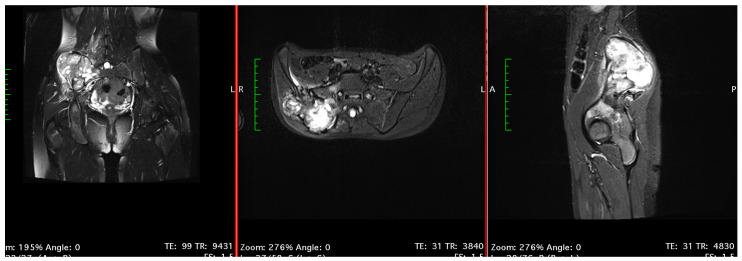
Osteosarcoma of the right ilium in MRI view.
